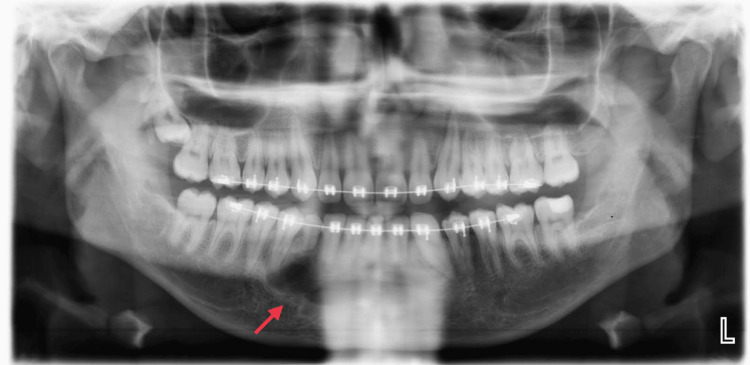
Panoramic radiograph after six months followed up.
